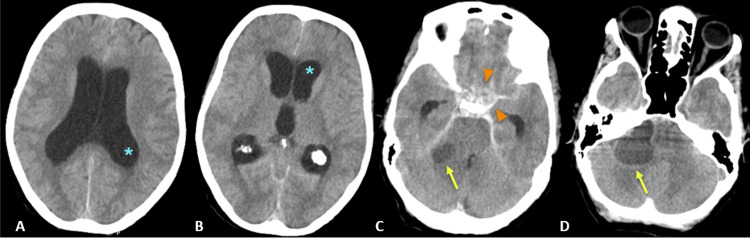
Axial cranial CT images on admission. (A, B) Images showing acute hydrocephalus (blue asterisks indicating dilated ventricles); (C) image revealing subarachnoid hemorrhage in basal cisterns (orange arrowheads) and a right cerebellopontine angle lesion (yellow arrow); (D) right cerebellopontine angle lesion (yellow arrow).
